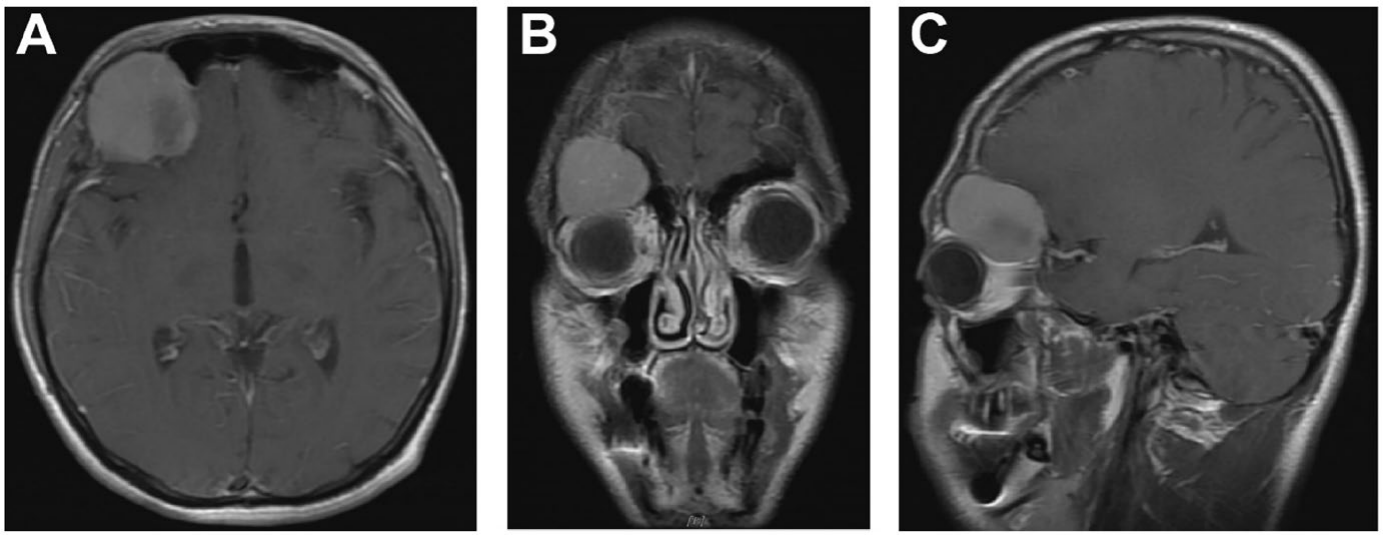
MRI characteristics of the fronto‐orbital osteoblastoma. (A) Axial postcontrast T1‐weighted image depicting the tumor exerting pressure on the right orbital roof. (B) Coronal T2‐weighted image revealing a homogenously hyperintense extra‐axial mass centered at the anterior cranial fossa‐orbital apex junction with inferior displacement of the globe. (C) Postcontrast sagittal T1‐weighted image showing vivid enhancement of the lesion.
